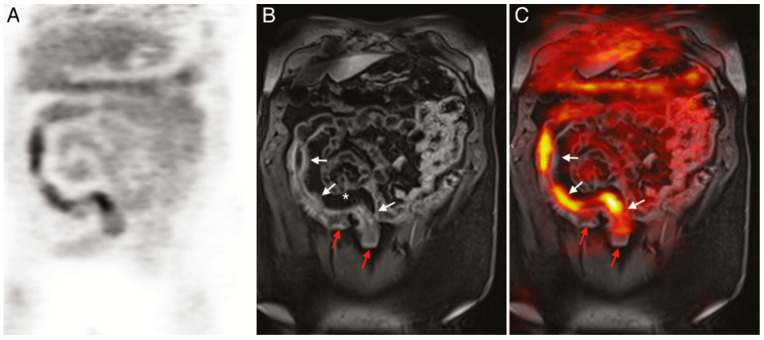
(A) ^18^F-FDG PET image of human patient with Crohn’s disease. (B) T1-weighted magnetic resonance imaging (MRI) image of the same patient. (C) Merged PET/MRI. White arrows indicate locations of acute inflammation while red arrows highlight damage resulting from earlier disease action. The asterisk (*) shows a site of proliferation of fibrofatty compounds in the mesentery. SUVmax of ^18^F-FDG 5.6–9.2 vs. SUVmax of background bowel 1.5–2.8 [147].
